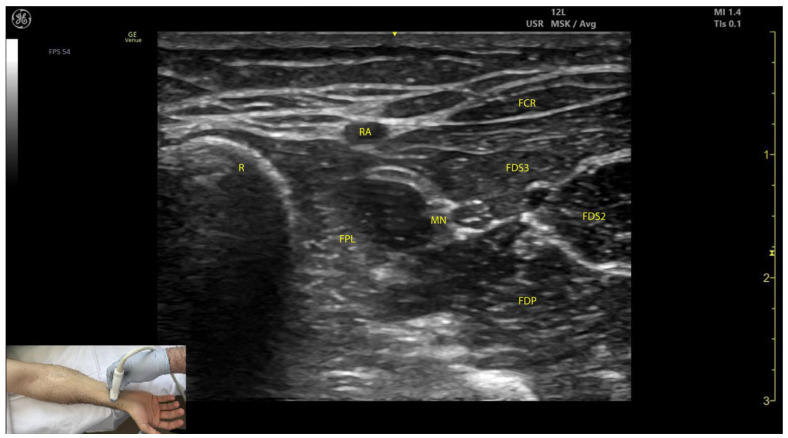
Ultrasound anatomy of the flexor pollicis longus (FPL) with key landmarks: FPL—flexor pollicis longus; R—radius; MN—median nerve; RA—radial artery; FDS3—flexor digitorum superficialis digit 3; FDS2—flexor digitorum superficialis digit 2; FDP—flexor digitorum profundus.
